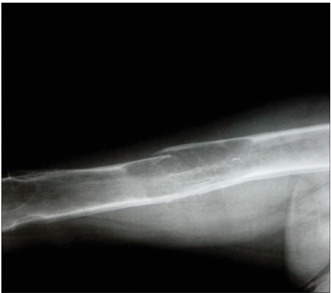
Femoral radiograph showing fragility fracture due to brown tumor in the femoral diaphysis; in the distal region of the femur, there are another two brown tumors, consisting of expansible well-defined lesions.
